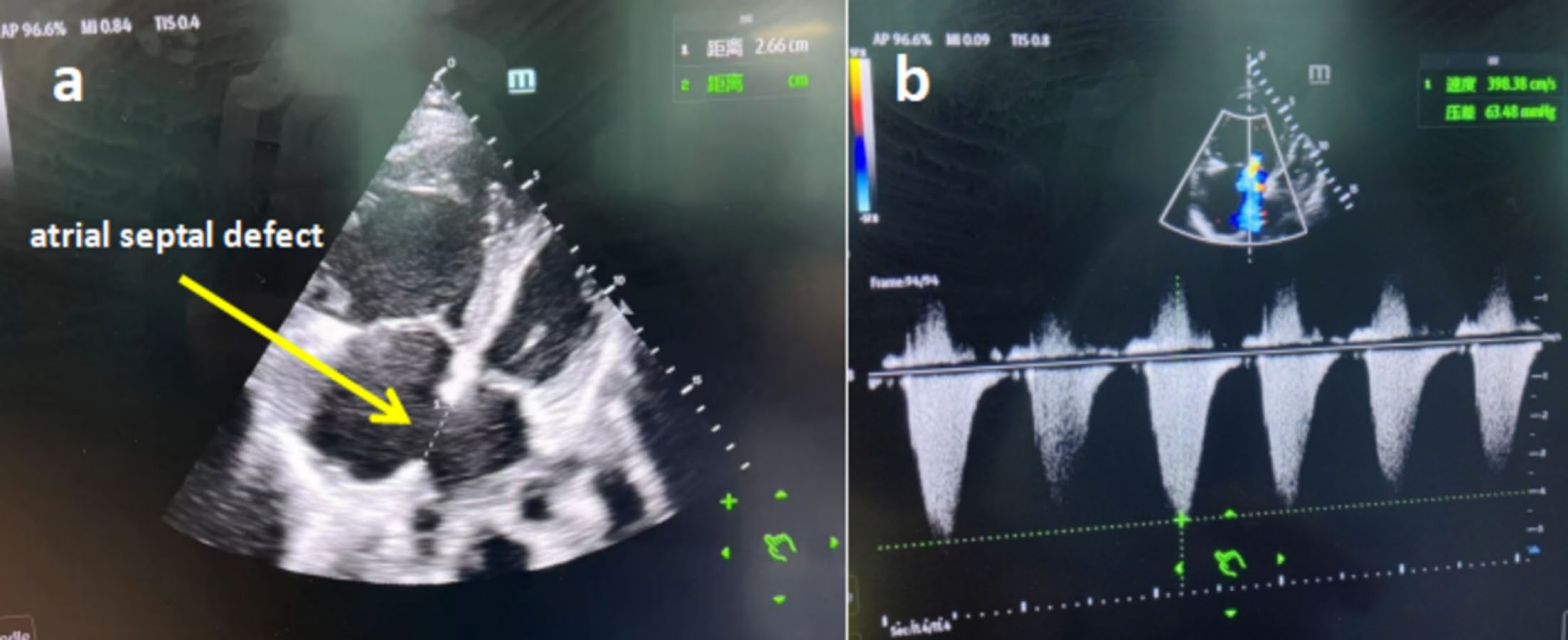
(a) Cardiac ultrasound showed the patient with severe atrial septal defect. (b) Cardiac ultrasound showed the patient with severe pulmonary hypertension.
